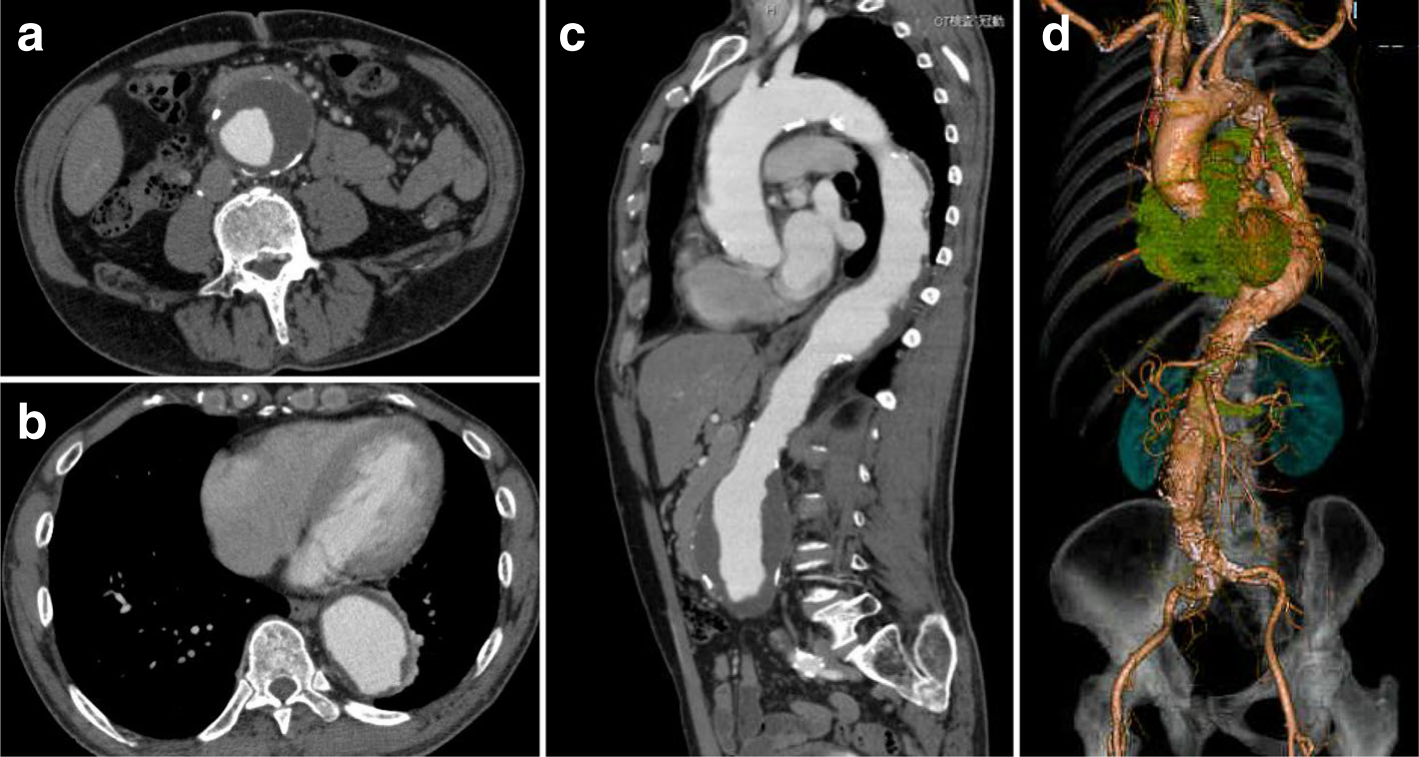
Contrast enhanced computed tomography of case 1 before the operation for abdominal and thoracic aortic aneurysm. a, b Axial view. a Aneurysmal dilatation with intraluminal thrombus was observed in the abdominal aorta. b The thoracic aorta was also enlarged. c, d Sagittal reconstruction (c) and 3-dimensional reconstruction (d), demonstrating the morphology of the thoracic and abdominal aorta and branches
